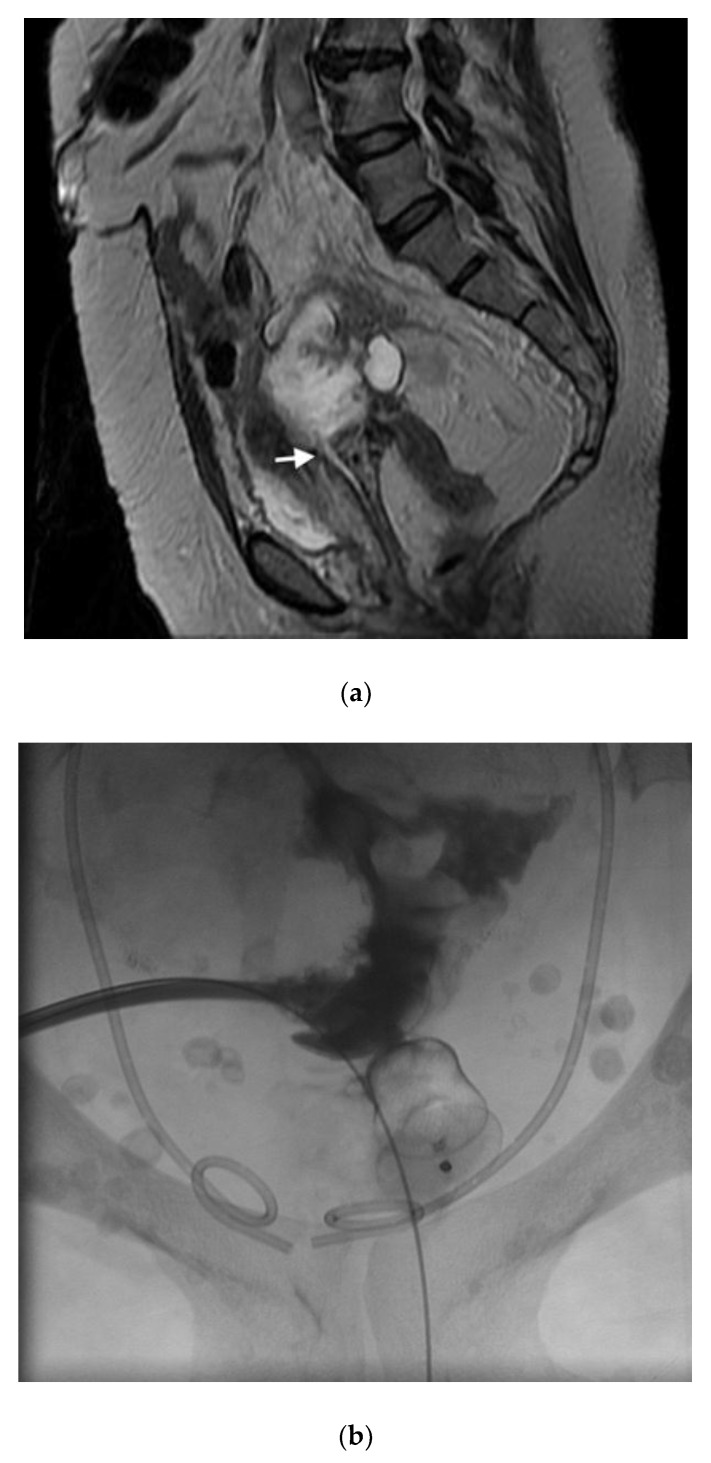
(a) Sagittal T2 MR imaging shows communication between a pseudomyxomatous tumor and the vagina (arrow); (b) contrast injection shows no further communication between the tumor and the vagina just after deployment of the Amplatzer 2 plug.
